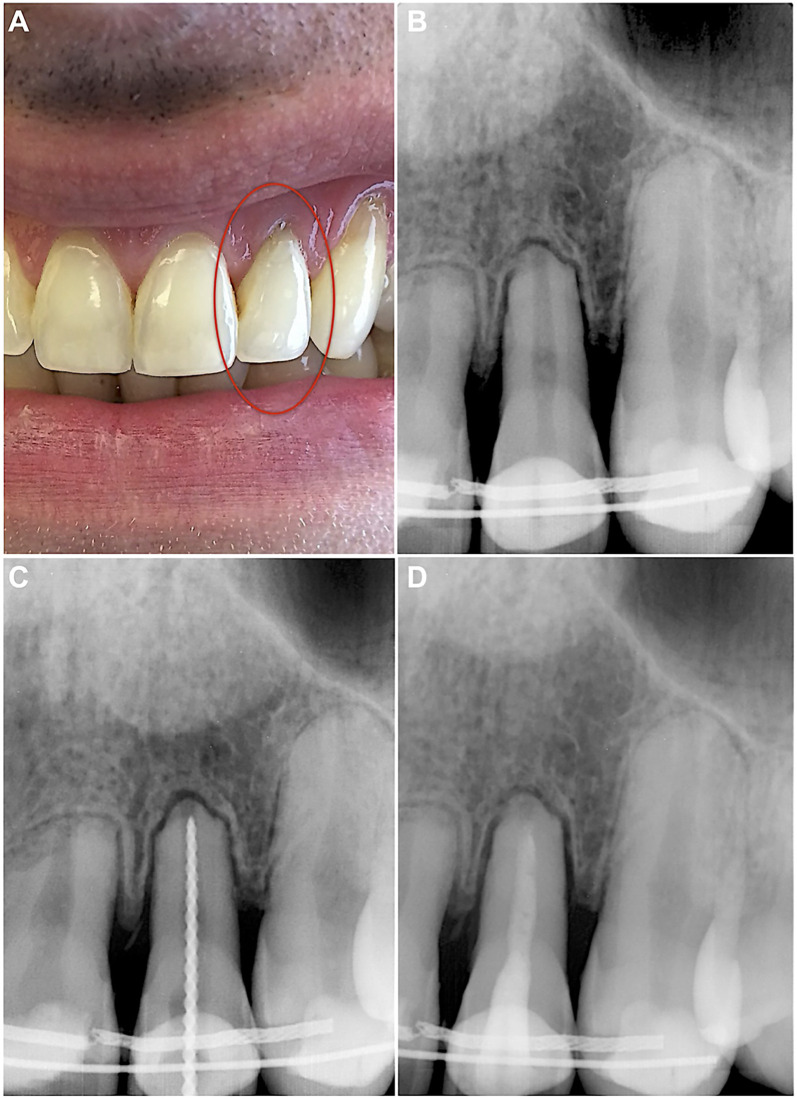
(A) Preoperative intraoral photo taken during the examination on the first visit. (B) Preoperative periapical radiograph revealing apical root resorption of teeth 21, 22, 23, and widened periodontal ligament of tooth 22. (C) Intraoperative periapical radiograph for WL determination with #45/0.02 K-File. (D) Postoperative periapical radiograph of cleaned and shaped root canal, filled with UltraCal XS paste. The access cavity was sealed with reinforced zinc oxide-eugenol restorative cement IRM.
